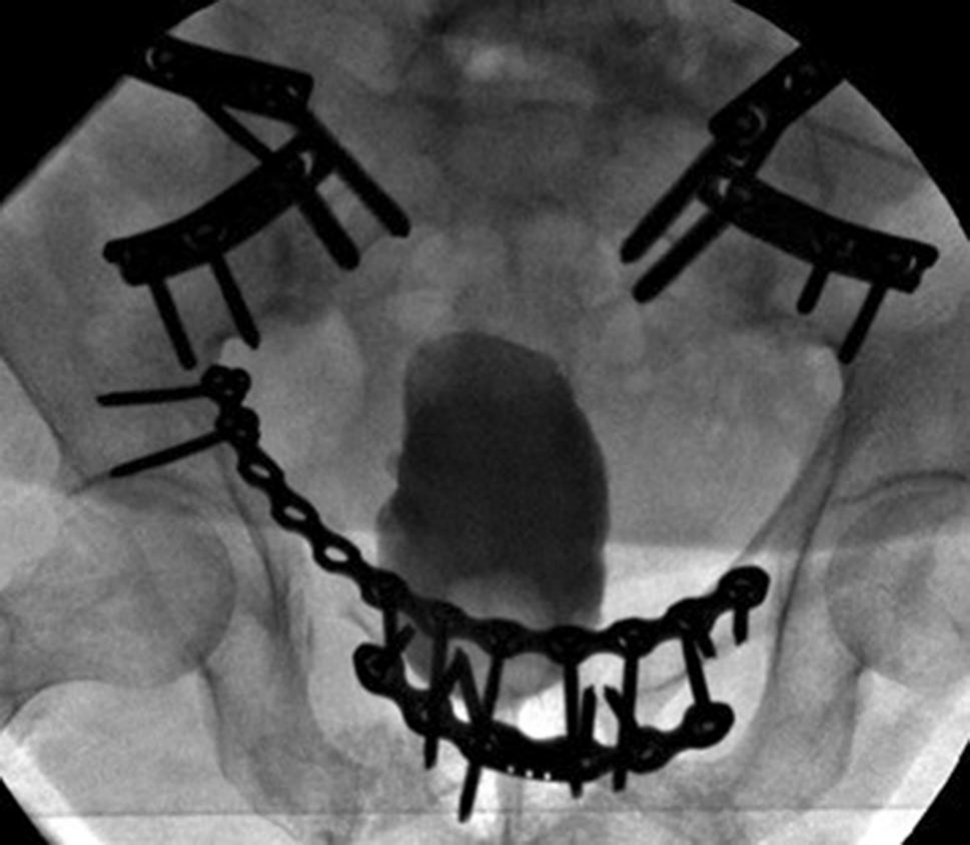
Intraoperative AP X-ray of the pelvic ring with cystogram. The bladder is back into its anatomical position
